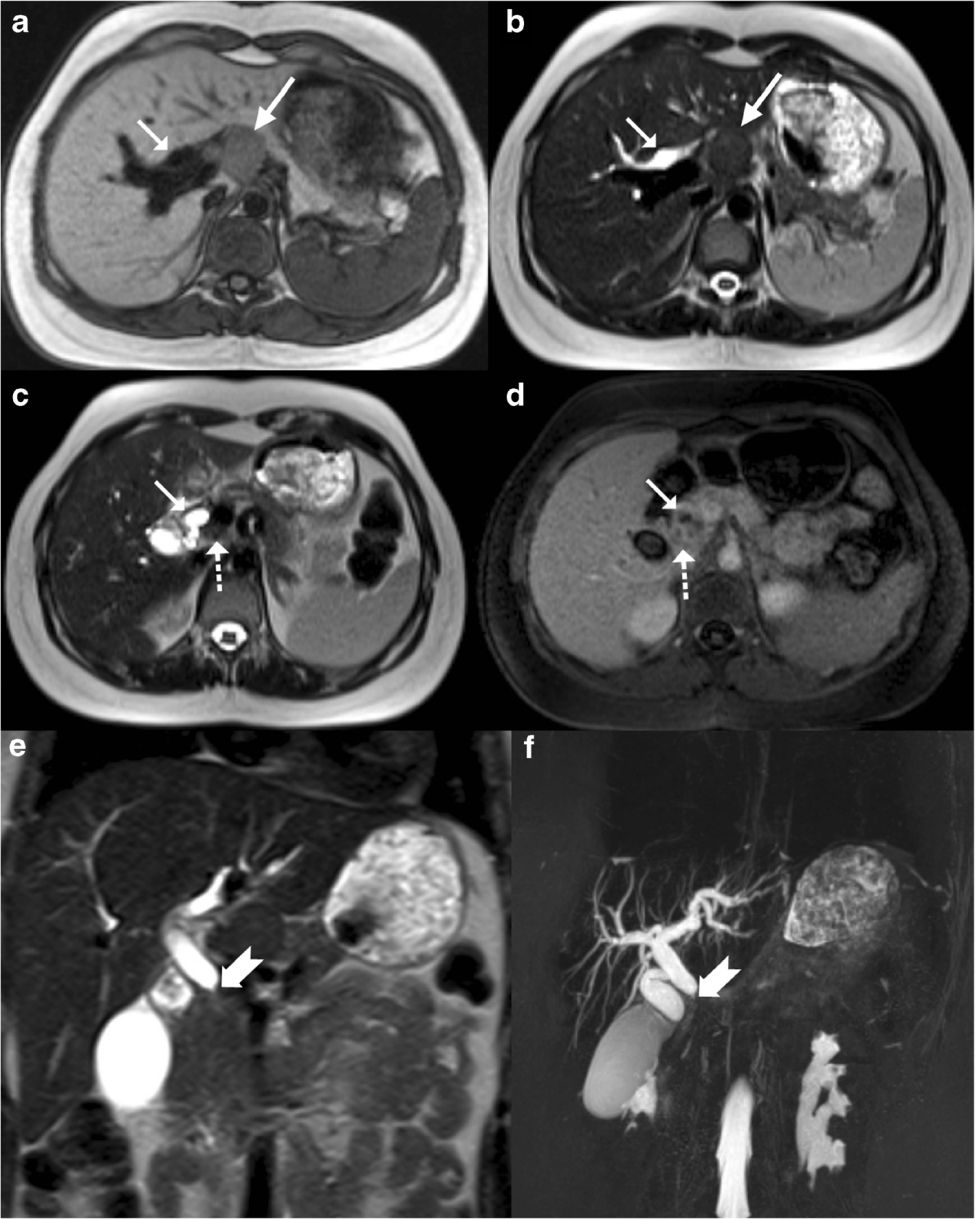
A 21-year-old young woman a known case of disseminated tuberculosis with obstructive jaundice. a, b Axial T1- and T2-weighted MRI display dilated biliary tree (*arrow*) with a non-necrotic nodal mass at the hepatic hilum (*long arrow*). c Axial T2-weighted MRI reveals a smaller node (*dotted arrow*) along the central bile duct (CBD; *arrow*), which is dilated. d Post-contrast T1-weighted image shows mild enhancement in the node as well as along the walls of the CBD. e, f Coronal T2-weighted MRI and thick-slab 3D MRCP beautifully delineates the tight narrowing of the mid extrahepatic common duct in this patient
